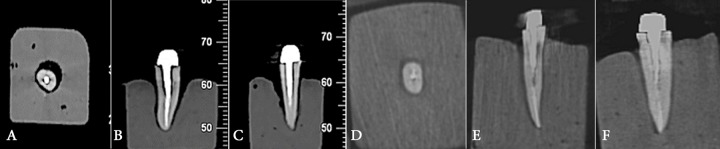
CBCT scans of teeth in New tom VGI system; *A)* Axial, *B)* Coronal, *C) *Sagittal and Scanora 3D; *D) *Axial, *E)* Coronal, *F)* Axial
